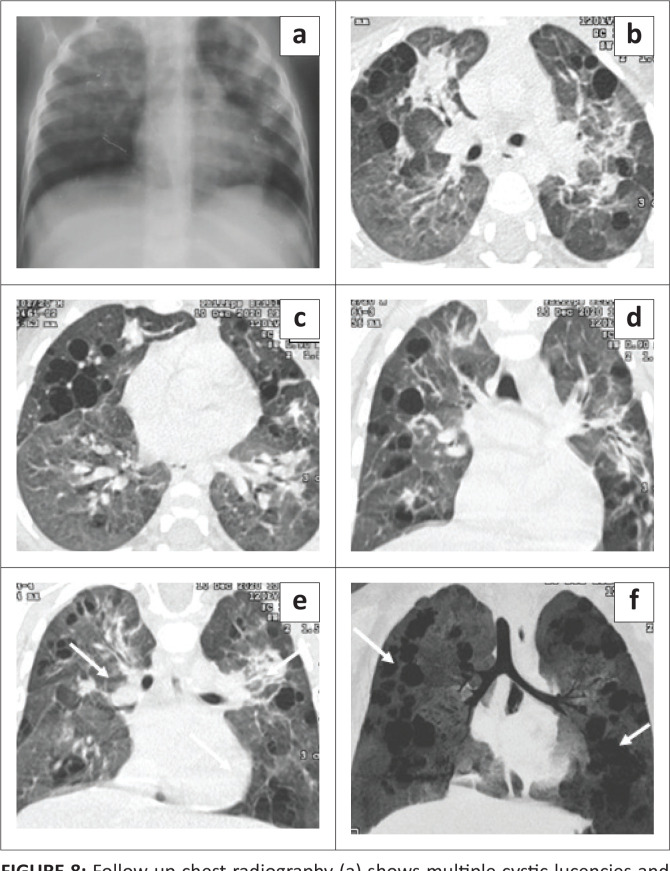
Follow-up chest radiography (a) shows multiple cystic lucencies and ill-defined opacities in both lungs, predominantly in the upper zones. Contrast-enhanced CT chest axial (b, c) and coronal (d, e) images reveal resolving consolidation with associated architectural distortion (arrows in e), traction bronchiectasis in the bilateral perihilar regions and multiple cysts of varying sizes in both lungs (arrows in f), better appreciated on the minimum intensity projection (MinIP) image.
